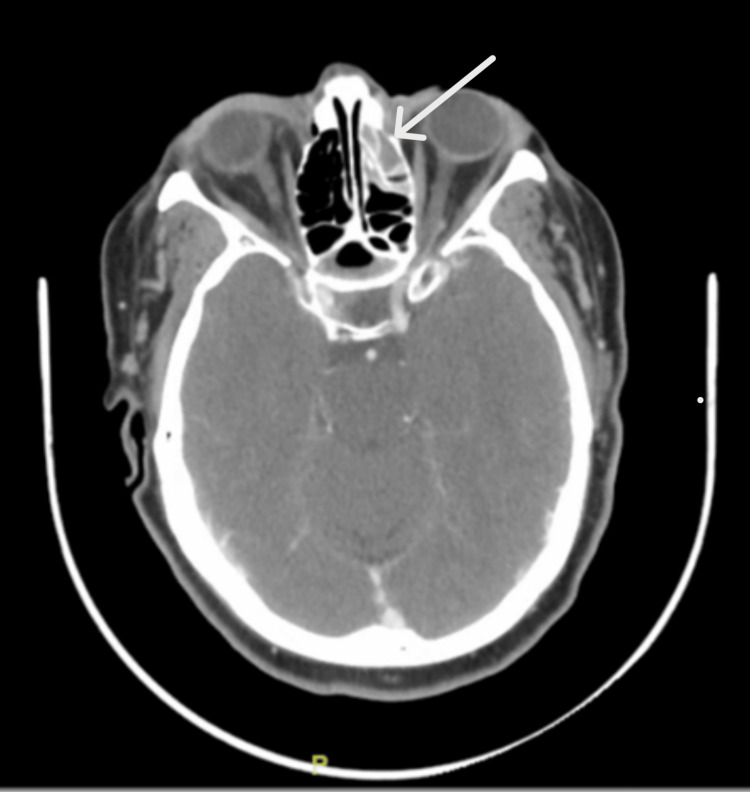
The axial section of the head CT showing opacification of the inferior aspect of the left frontal sinus and left sphenoid sinus (marked by the white arrow)
